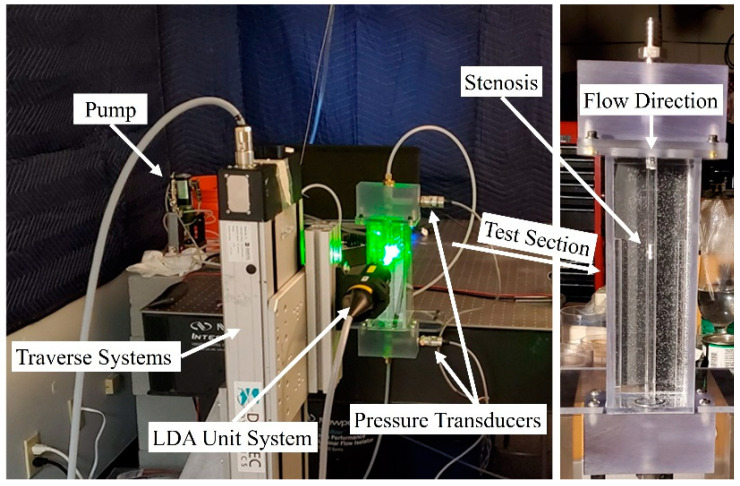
Experimental setup of the Laser Doppler Anemometry (LDA) axial velocity measurements for a constricted pipe representing arterial stenosis.
